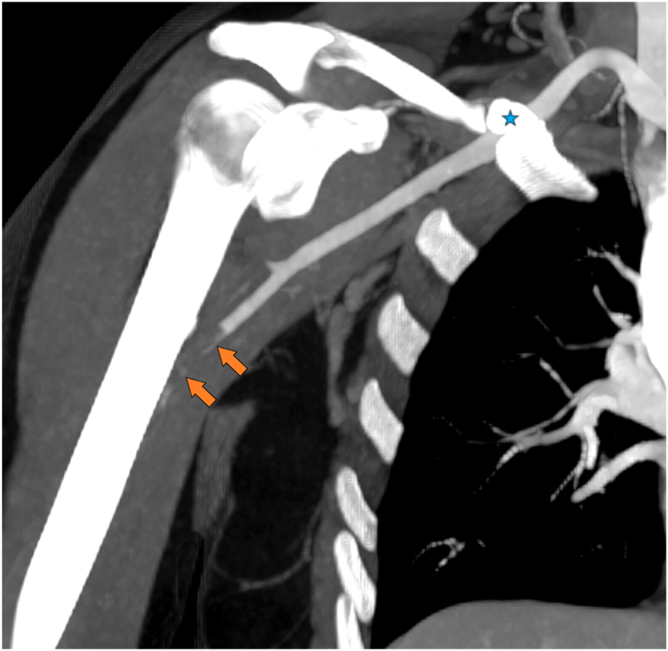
Coronal MIP CTA image showing complete thrombotic occlusion (*orangearrow*) at the axillohumeral arterial junction and, more proximally, a hypertrophic clavicular pseudarthrosis overlying the subclavian artery (*blue star*). *MIP*, Maximum Intensity Projection; *CTA*, computed tomography angiography.
